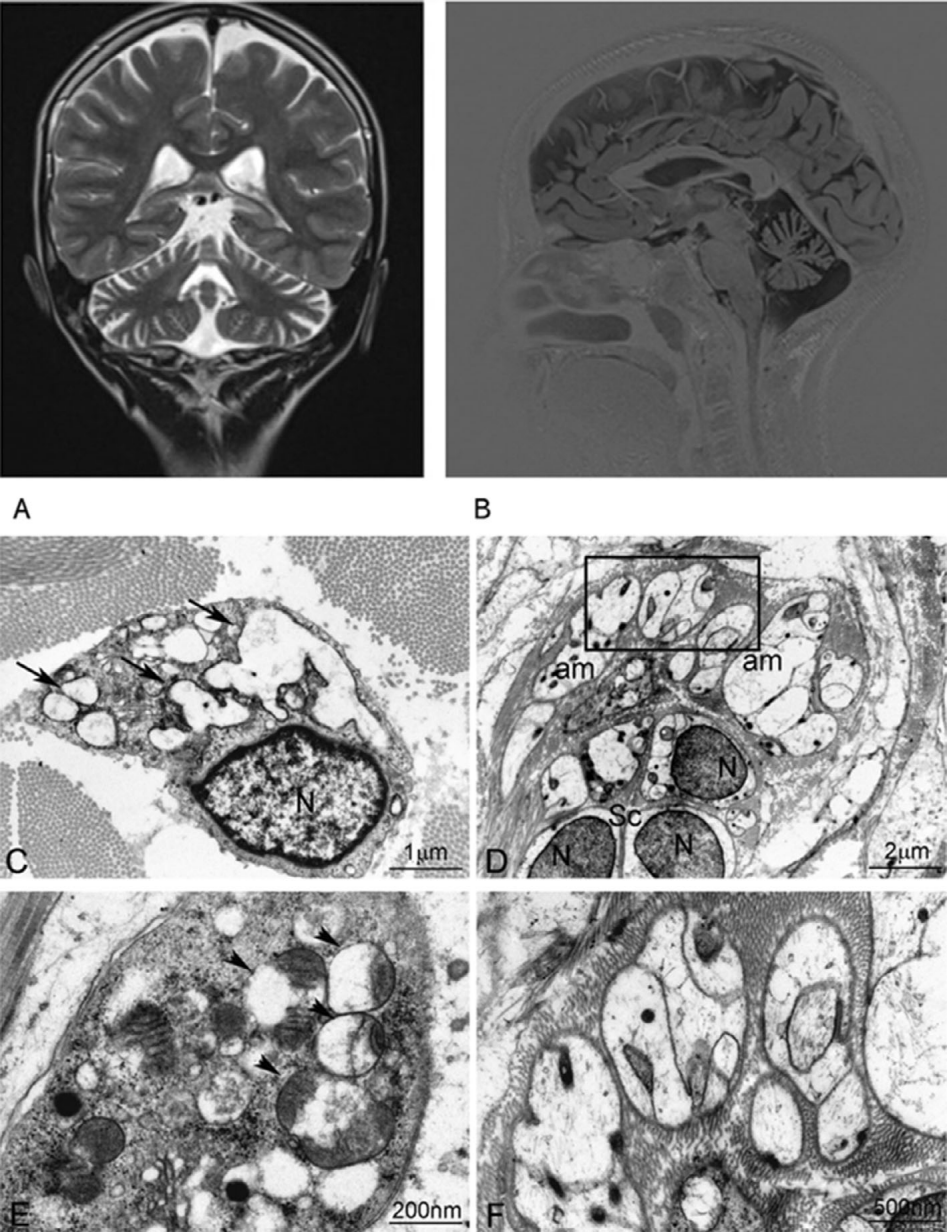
Brain MRI, coronal (A), sagittal (B); Ultrastructural analysis of skin biopsy. Swelling of the rough endoplasmic reticulum (arrows) in dermal fibroblasts (C), with mitochondrial alteration (arrowheads) (E); amyelinated nervous fibers show axon swelling at low (D) and higher magnification (F). N, nucleus; am, amyelinated nervous fibers; Sc, Schwann cells.
